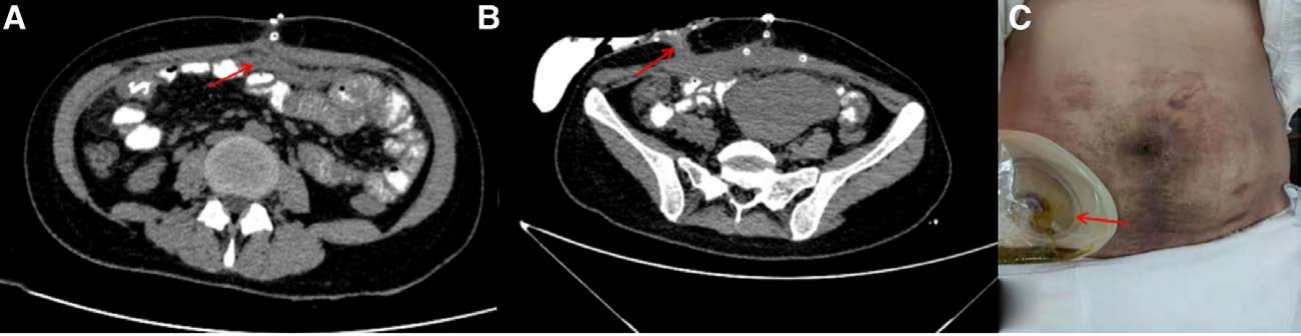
Stoma position of right lower abdomen, small intestine showed postoperative changes. (A, B) Reexamination of whole abdominal computed tomography (CT) after operation. (C) Body surface image.
